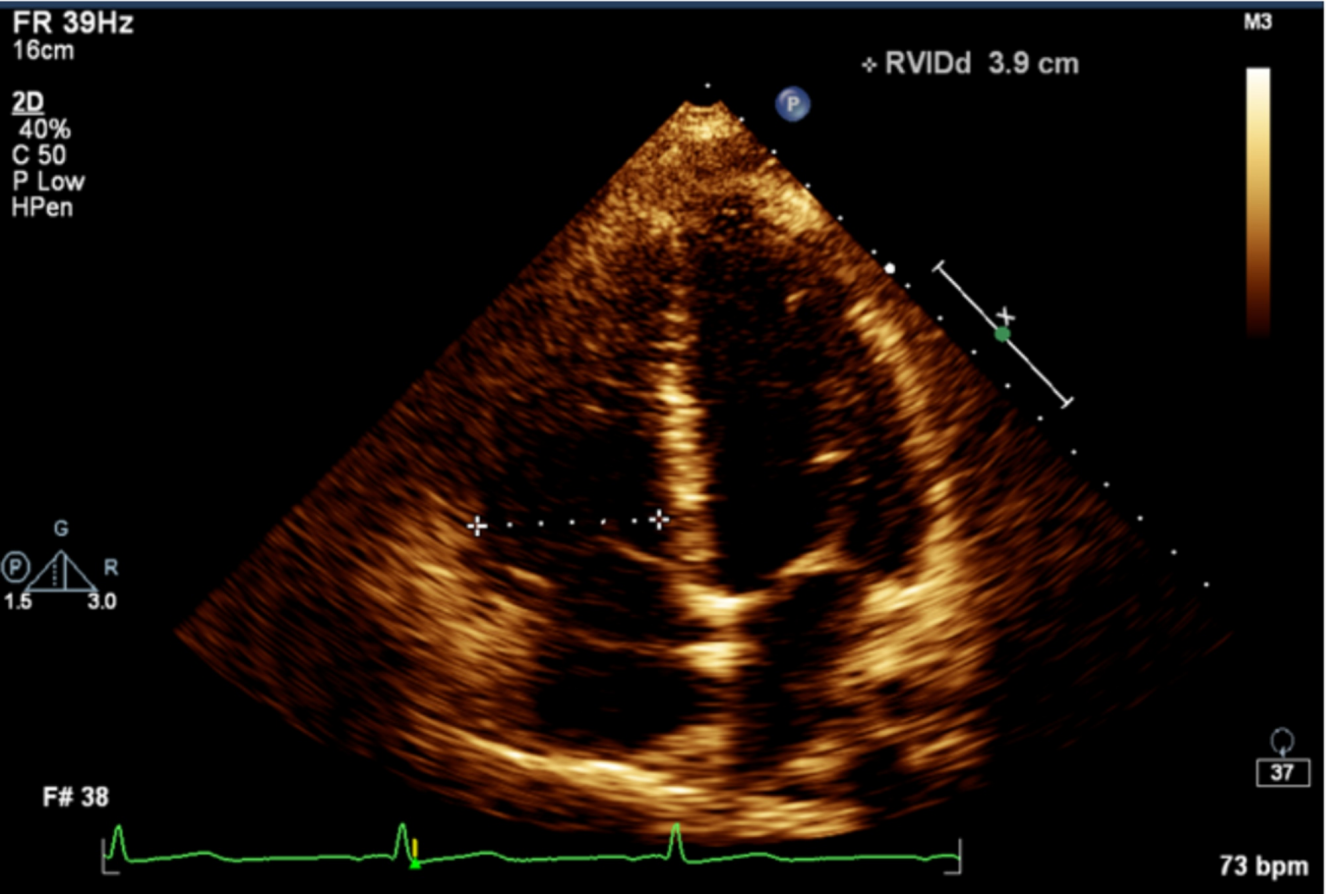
Right ventricular strain as demonstrated by enlarged right ventricle (shown by dashed line) on a four-chamber view of echocardiogram.
